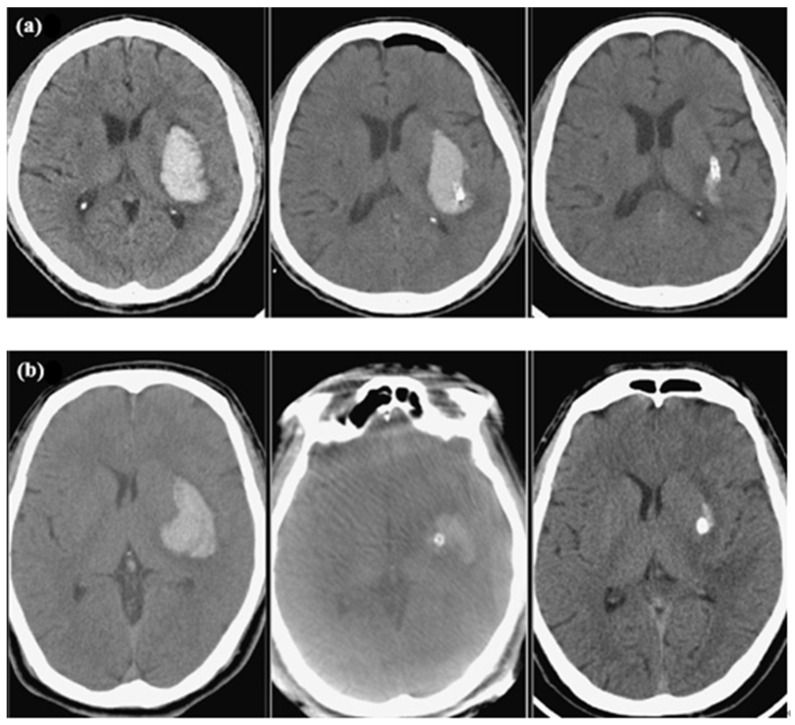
CT scans showing examples of left ganglionic ICH patients at baseline presentation (left), after aspiration with catheter placement (middle), and in a post-fibrinolytic state (right). All scans demonstrate the largest size of the hematoma on axial images. The procedure was conducted using either Brainlab navigation (a) or syngo iGuide needle guidance (b).
